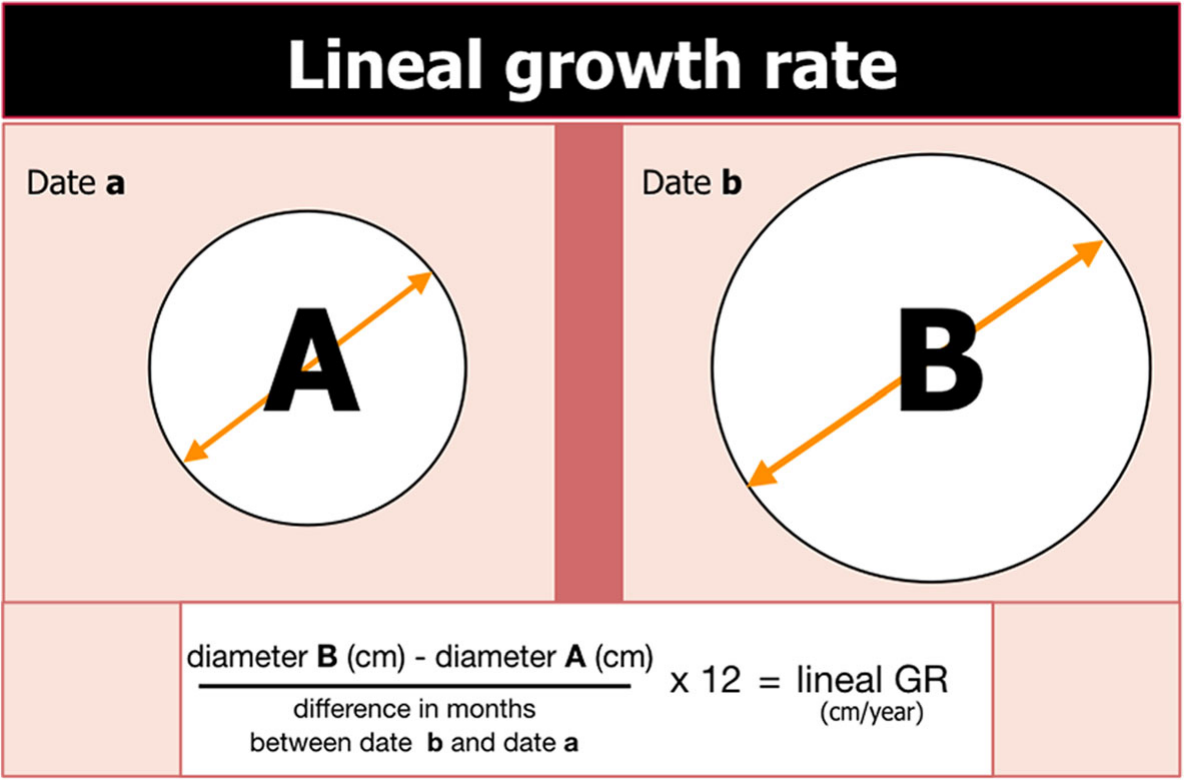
Formula of linear growth rate (GR) per year. To define the linear GR per year, divide the difference between the maximum tumor diameter (MTD) into two time points by the difference of the number of months between the two CT times and then multiply by 12
